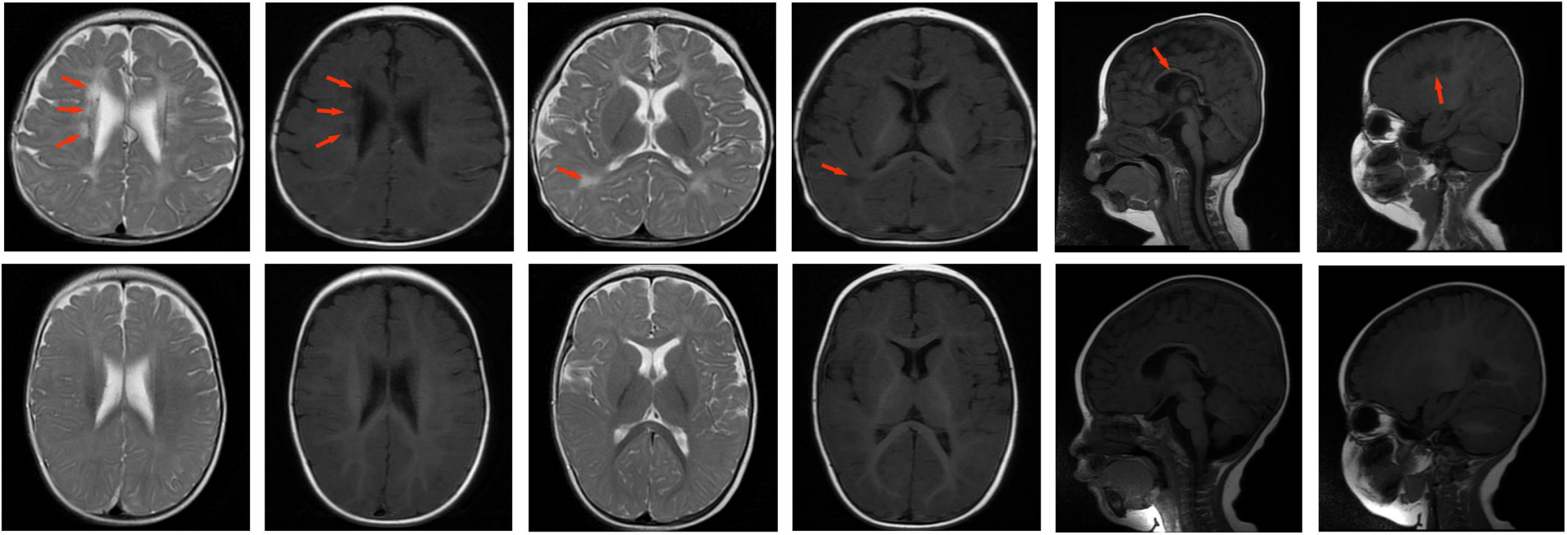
MRI scan of the patient's brain showed abnormal signal changes in the deep white matter area near the lateral ventricle and a mild delay of myelination compared with a healthy child at the age of 7 months
